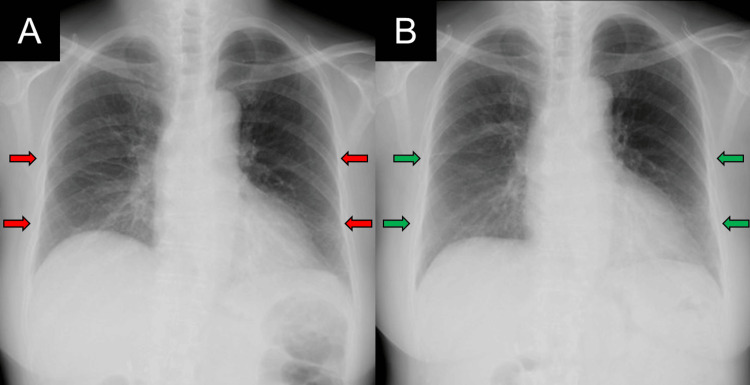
Chest radiographs on admission (A) and at two months after combination therapy (B) (A) Chest radiograph on admission showing bilateral diffuse ground-glass opacities (GGOs, red arrows). (B) Chest radiograph at two months after combination therapy showing improvement in lung volumes and GGOs (green arrows).
